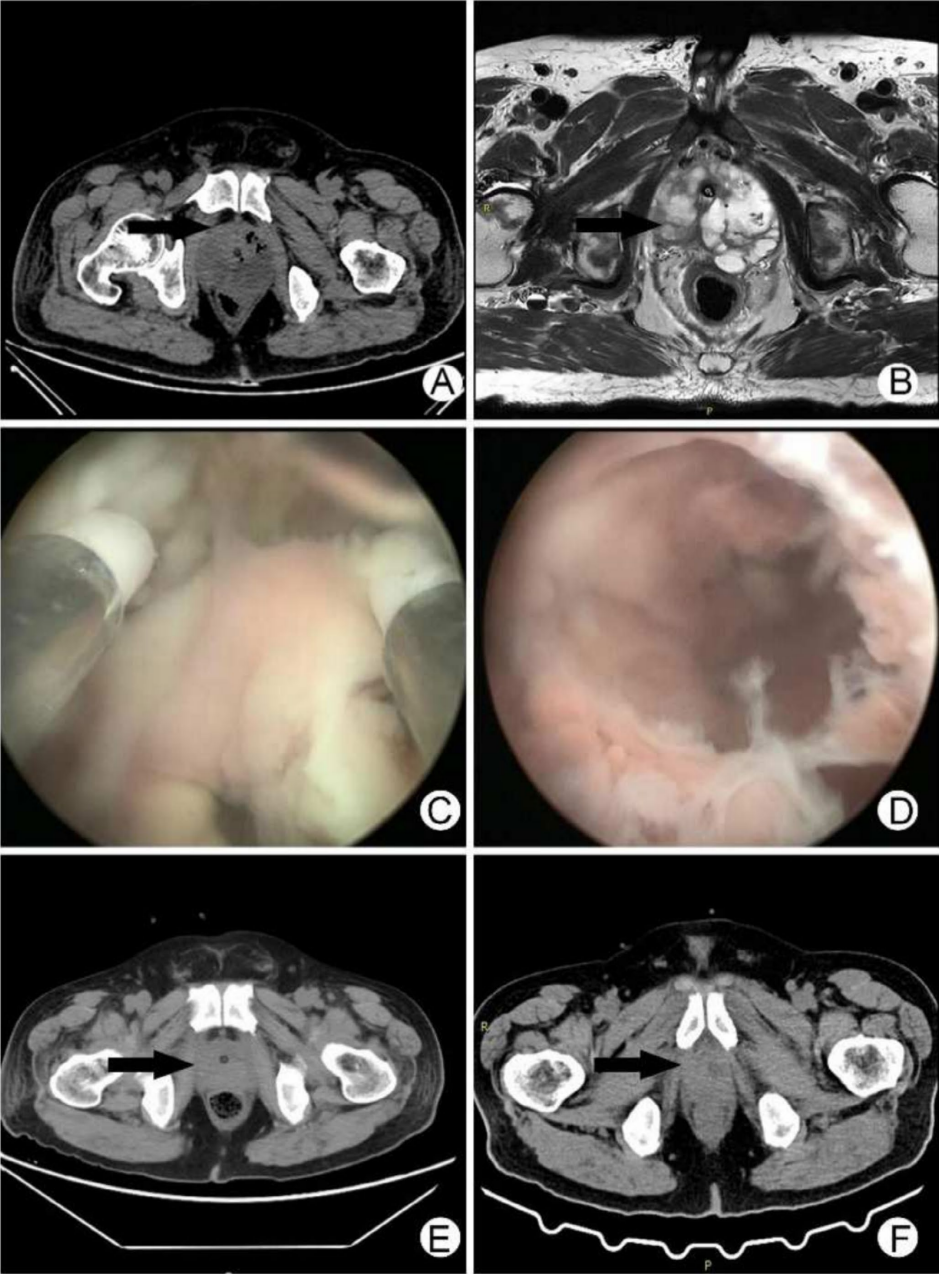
(A) Preoperative CT of the patient showed low‐density prostatic abscesses and intraprostatic gas (arrow position); (B) Preoperative MR of the patient showed prostatic abscess (arrow position); (C) Pink pus was found in the transurethral internal prostatic abscess incision; (D) Large pus of the prostate was found during transurethral internal resection of prostatic abscess; (E) Follow‐up CT performed 2 days postoperatively showed that the prostatic abscess subsided and no intraparenchymal gas (arrow position); (F) Follow‐up CT performed after catheter removal 2 weeks postoperatively showed that the prostatic abscess subsided and no intraparenchymal gas (arrow position).
